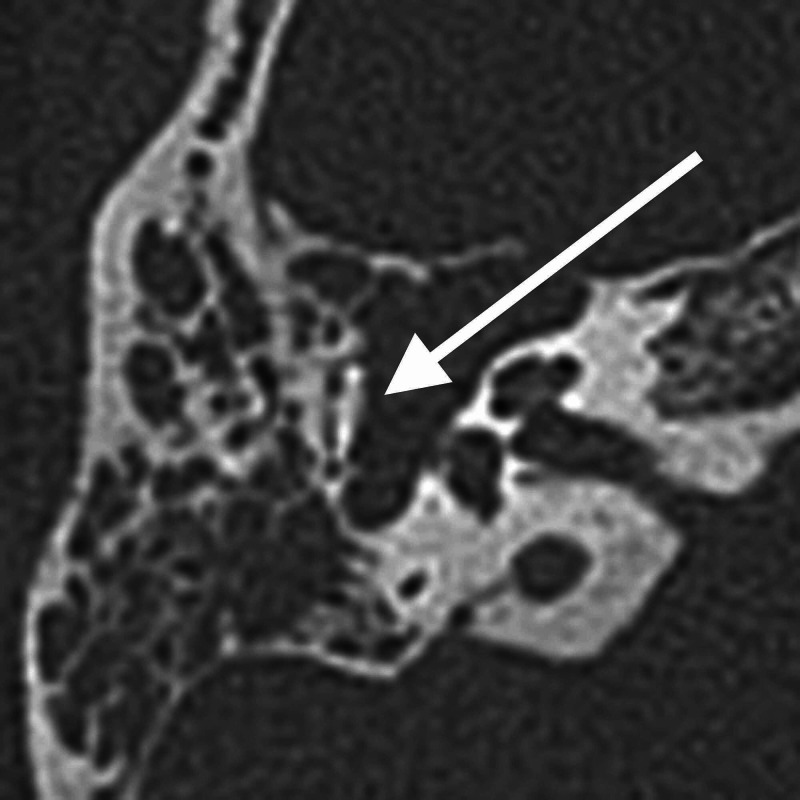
Axial view of HRCT of the right temporal bone The lesion is displacing the malleus and incus superolaterally. Note the erosion at the incudomalleolar joint (white arrow). HRCT - high-resolution computed tomography
